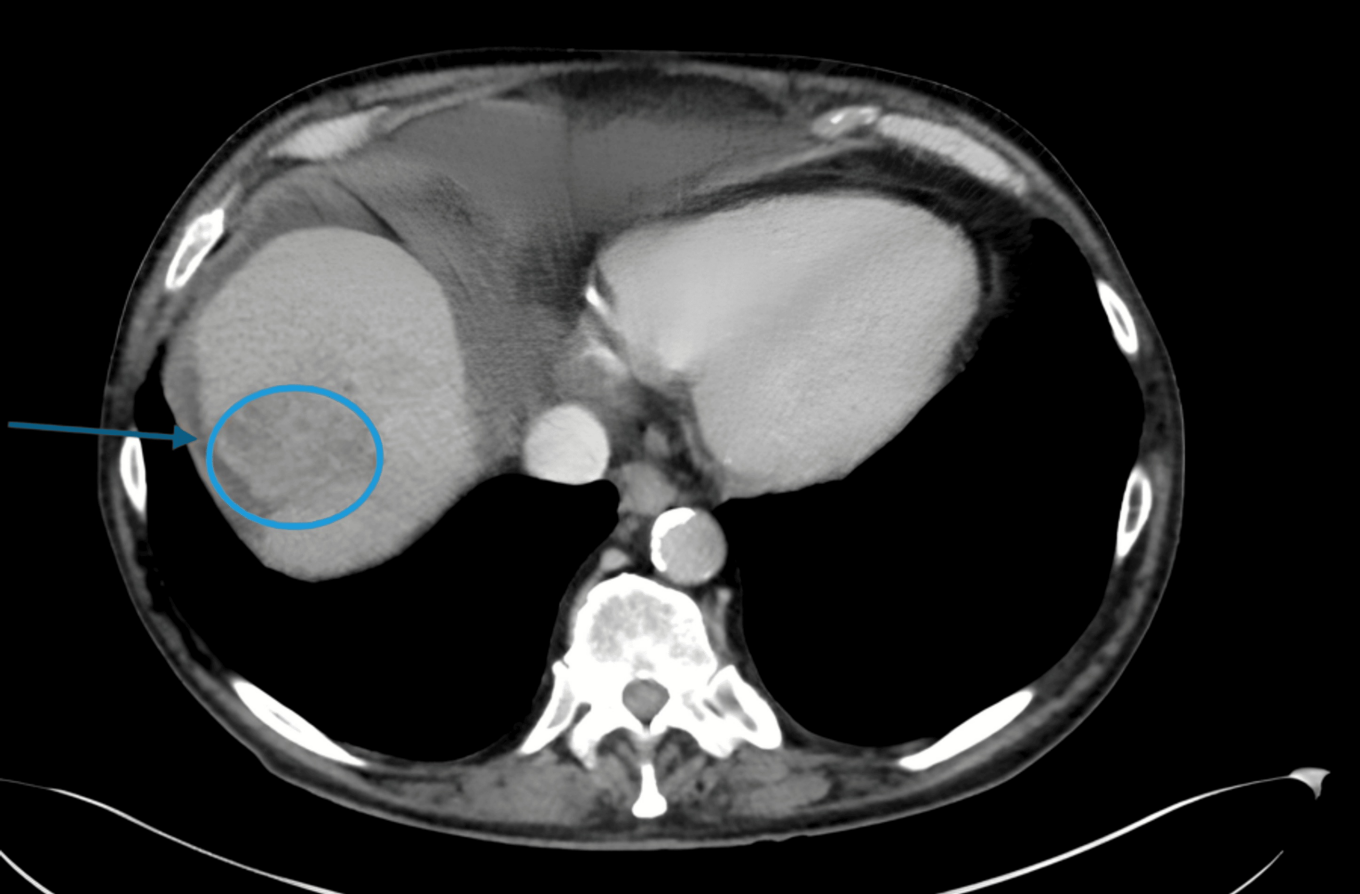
Contrast-enhanced abdominal CT scan (arterial phase) revealing a large 67 mm heterogeneous mass in liver segment VIII (indicated by blue circle and line), consistent with hepatocellular carcinoma in a dysmorphic liver CT: computed tomography
